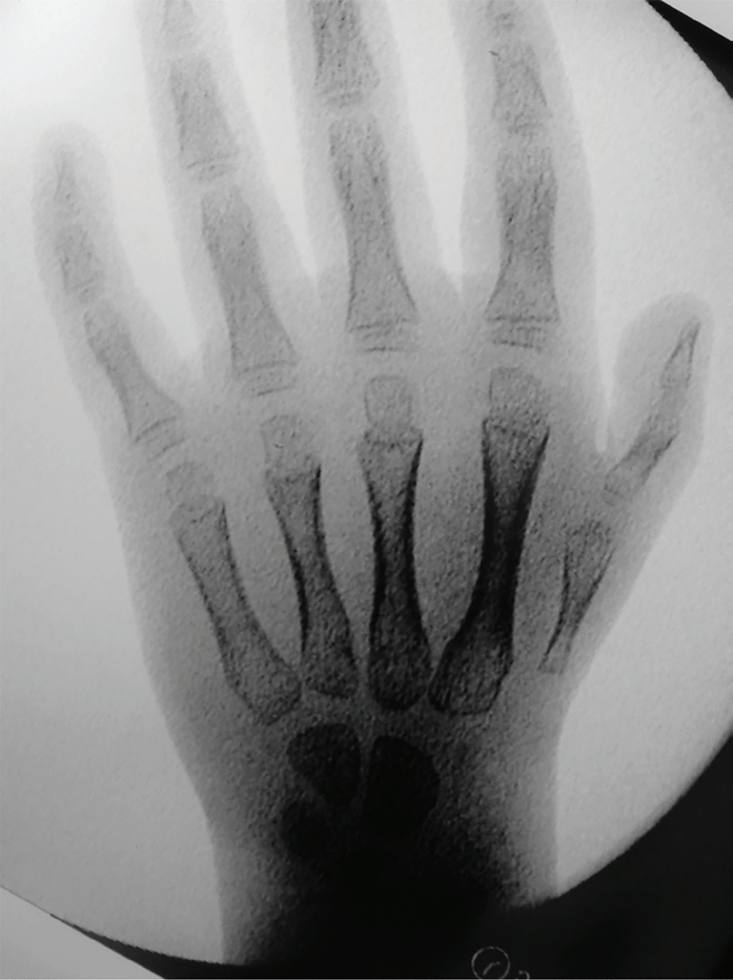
AP intra-operative x-rays demonstrate aplasia of the radial portion of the carpus with a hypoplastic first metacarpal
